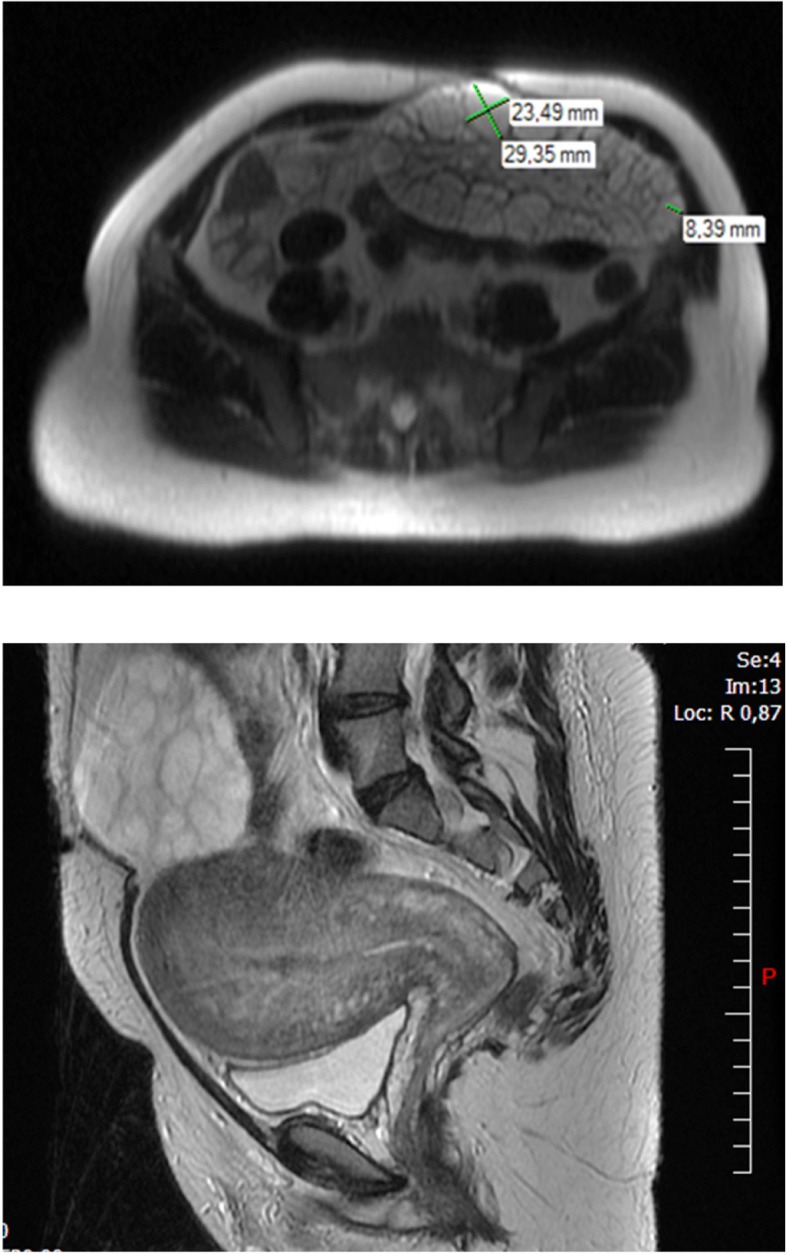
Pelvic MRI performed one month postpartum, left ovary (Case 1)
